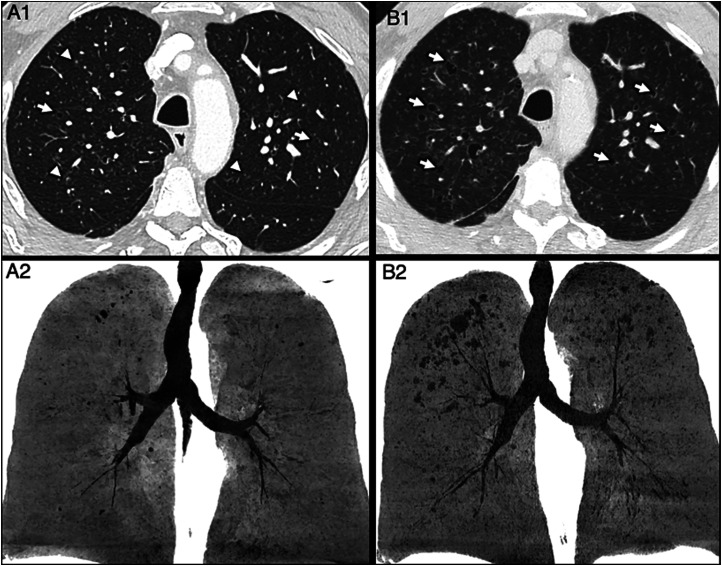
Axial contrast-enhanced CT images of the chest with lung windowing showing small cystic lesions in the initial chest-CT (arrows A1), which can be seen in the upper lung on a minimum intensity projection (MinIP) (A2). On a follow up scan 2 months later, these lesions can be seen to have grown in size and in number (arrows, B1). This is also seen on the MinIP image (B2).
